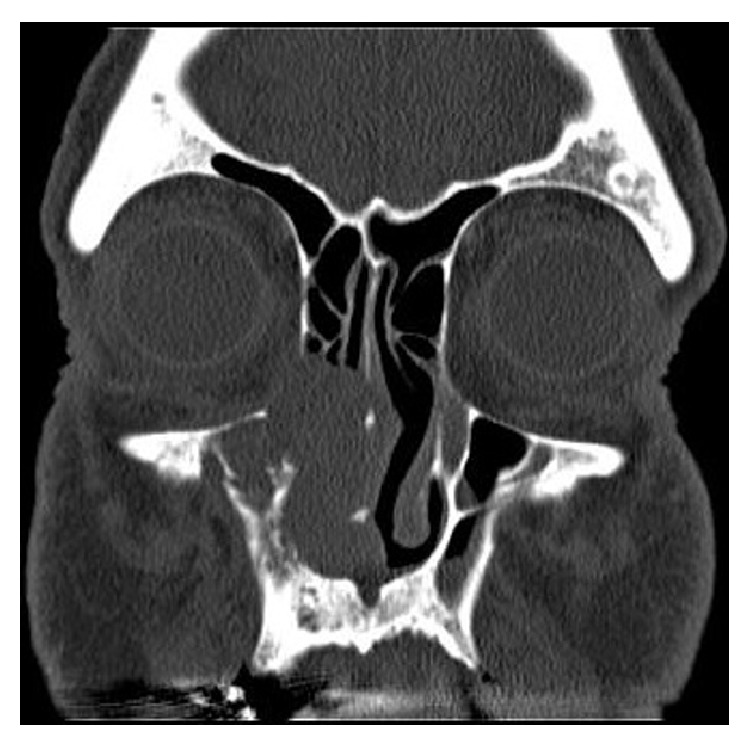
Coronal CT of the sinuses reveals right nasal maxillary mass with medial orbital wall destruction.
